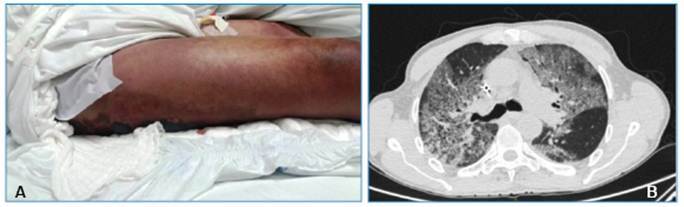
A: Hematoma on the right thigh. B: Chest CT showing ground-glass opacities in both lungs.
